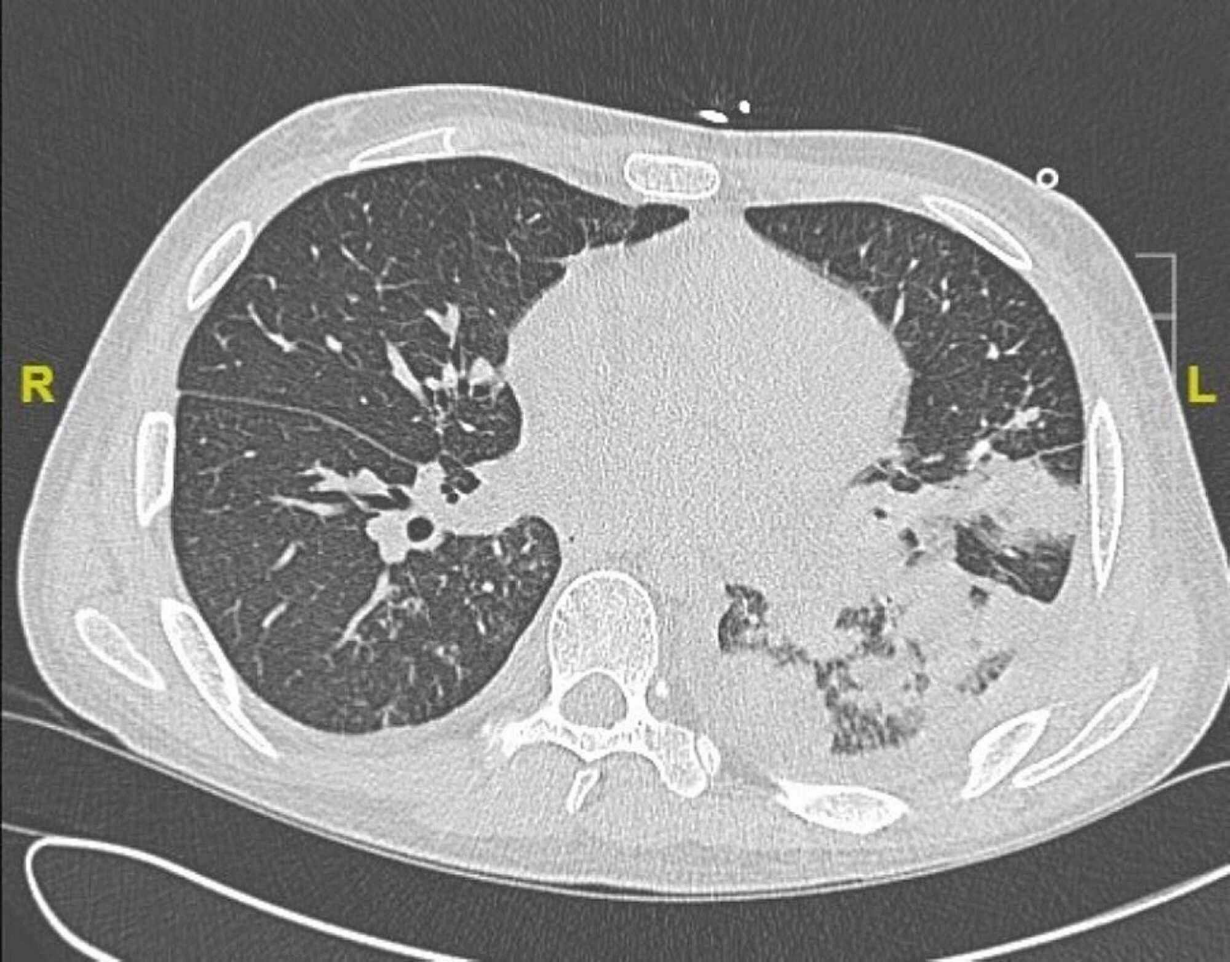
CT chest on admission CT chest obtained on admission to the hospital demonstrating nodular consolidation in the posterior left lower lobe of the lung.
